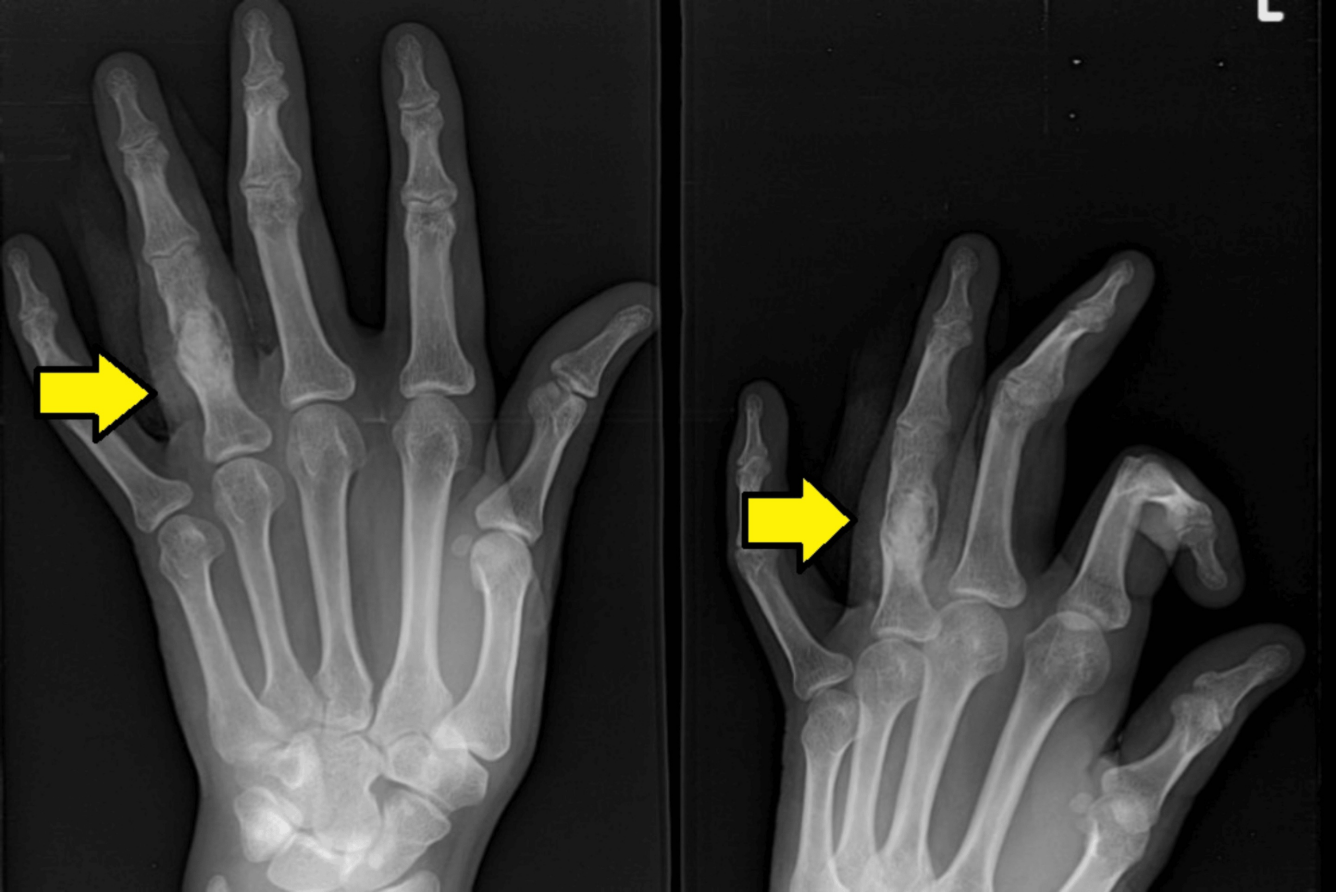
X-ray of the left hand showing sclerotic margins and a central diaphyseal lytic lesion in the proximal phalanx of the fourth finger (arrows)
